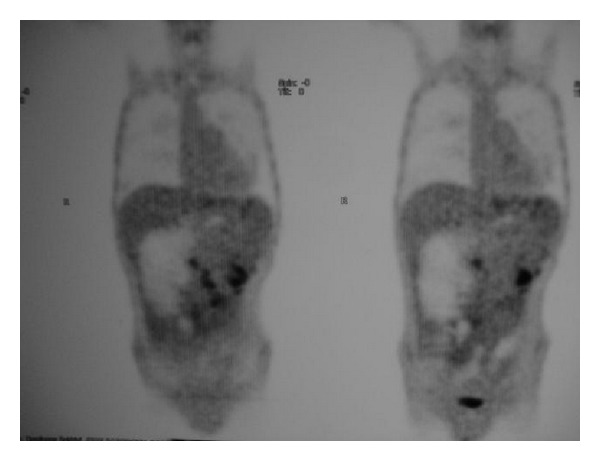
PET-CT showed peritoneal carcinomatosis in coronal reconstruction.
